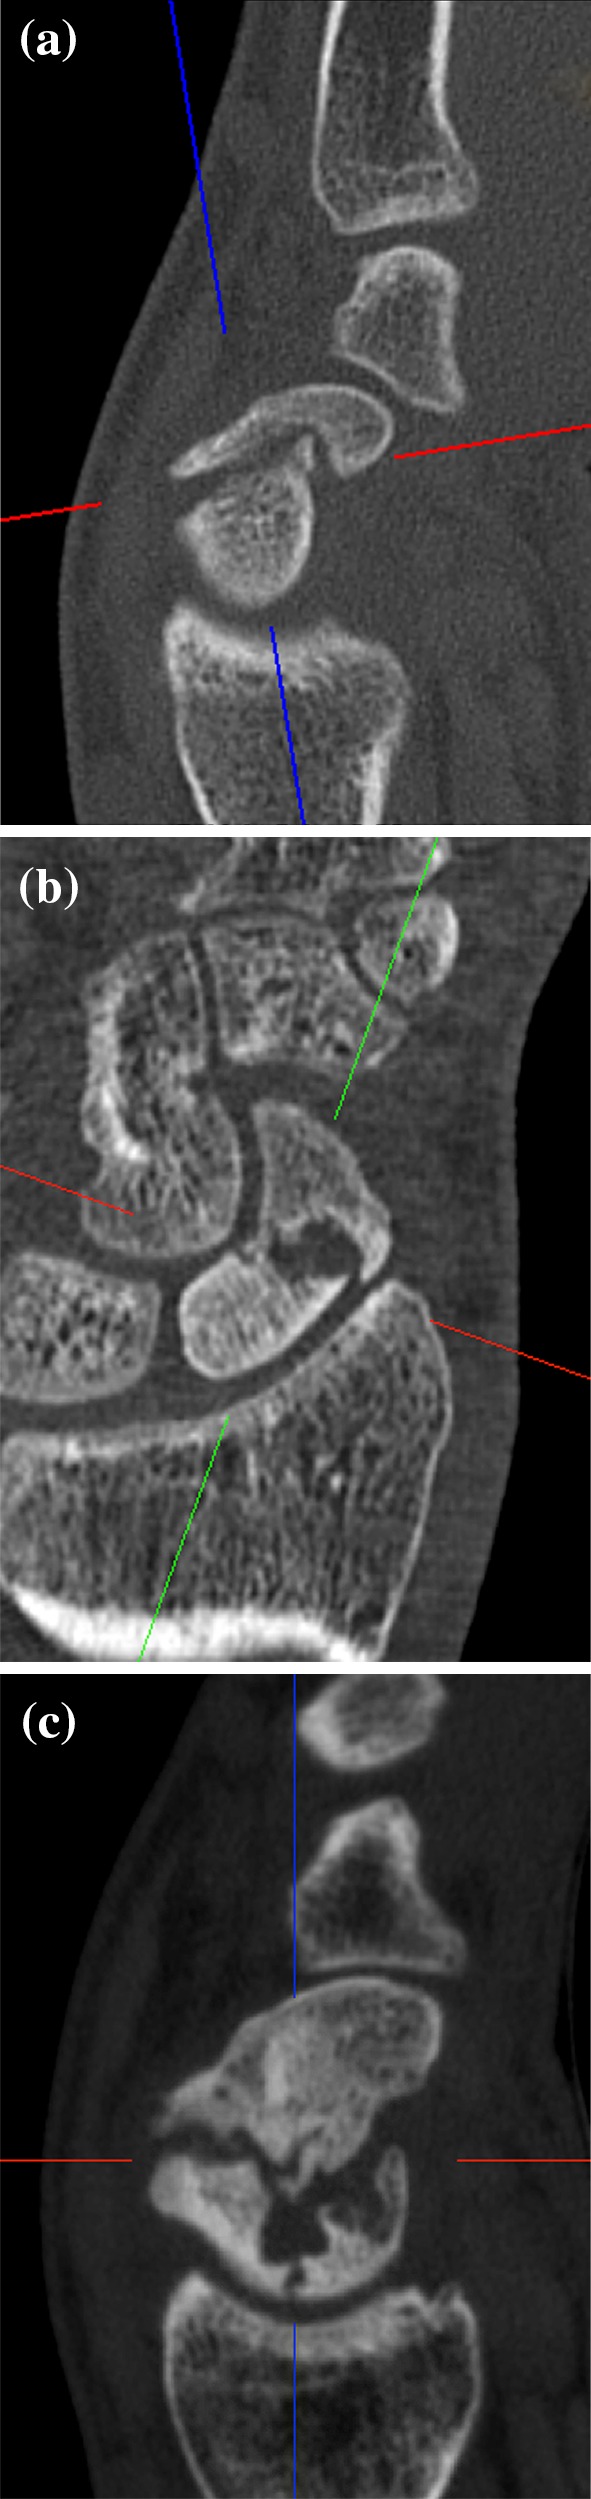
2D-CT parameters of the proximal fragment. a Trabecular bone structure. b Sclerosis of the proximal fragment in comparison to the distal fragment. c Fragmentation of the proximal pole
